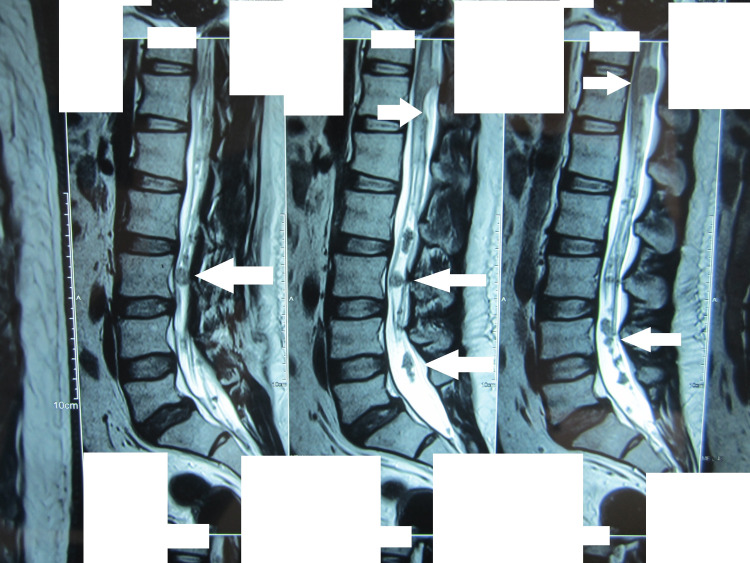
MRI of the lumbosacral region without contrast in T2 sequence showing multiple tumors compressing the spine in the lower thoracic and lumbar regions (arrows) MRI: magnetic resonance imaging
